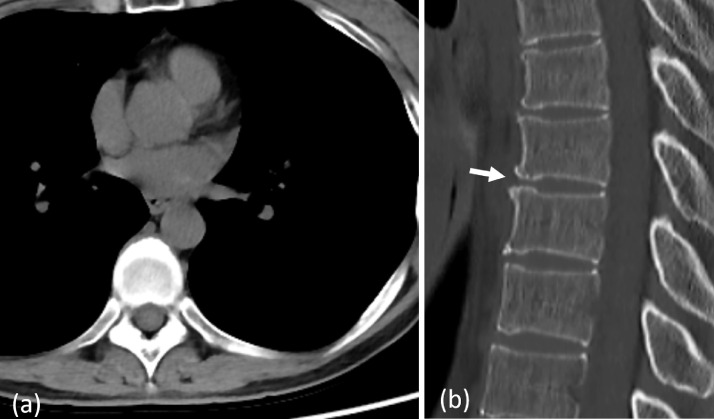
Computed tomography images of the thoracic spine 4 months later. (a) The mass is not observed. (b) The high density on the ventral side of the Th8-9 vertebral bodies are not visible (arrows).
